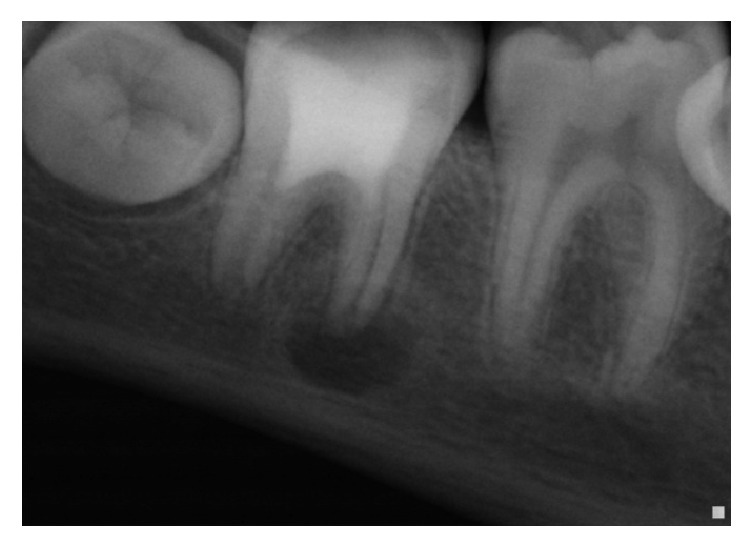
Radiograph after 10 months' recall: enlargement of the radiolucent image at the mesial roots.
